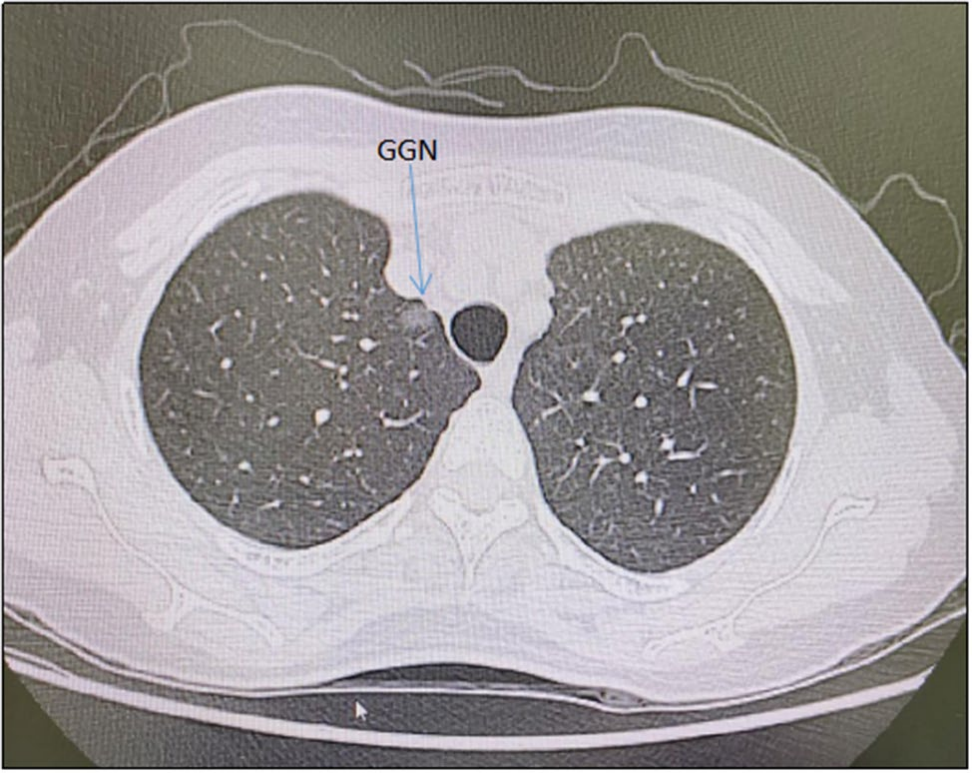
The preoperative chest computed tomography (CT) scan revealed a ground glass nodule (GGN) measuring 10 mm in diameter, located near the pleura of the right upper lobe
